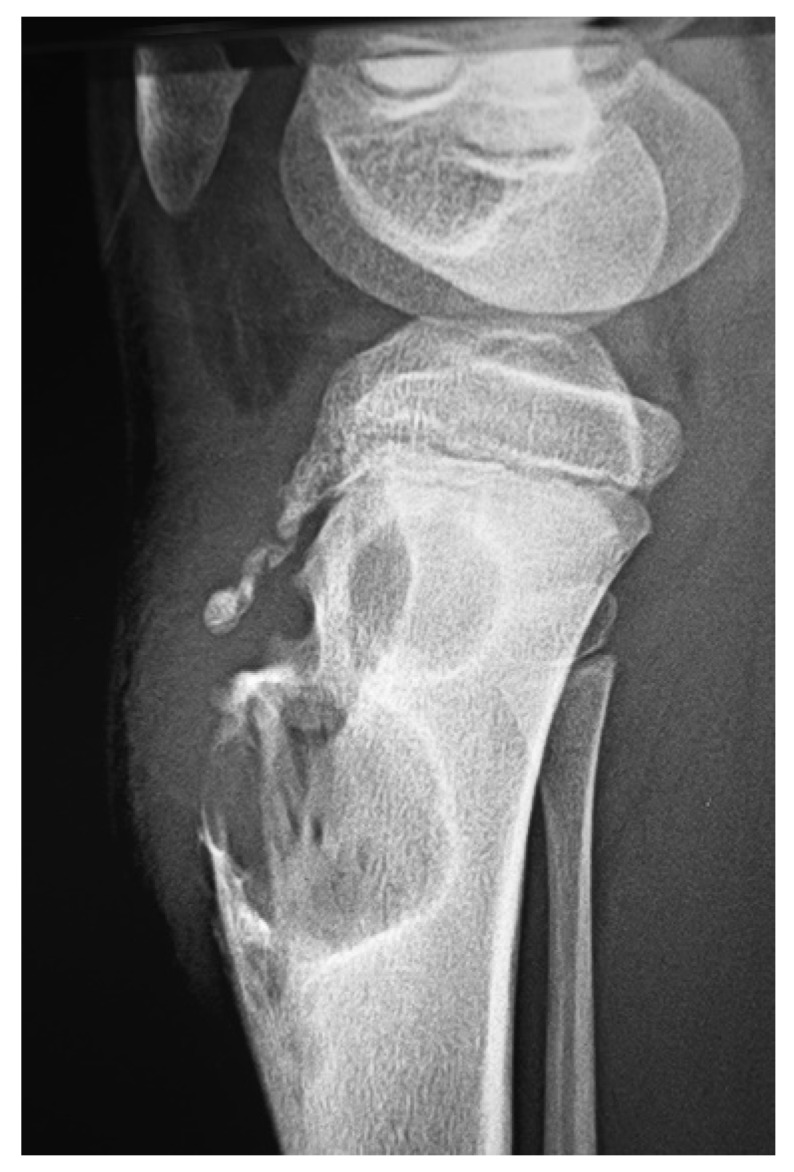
X-ray of thigh and knee eumycetoma showing massive soft tissue shadow, periosteal reaction and multiple bone cavities resembling osteogenic sarcoma.
